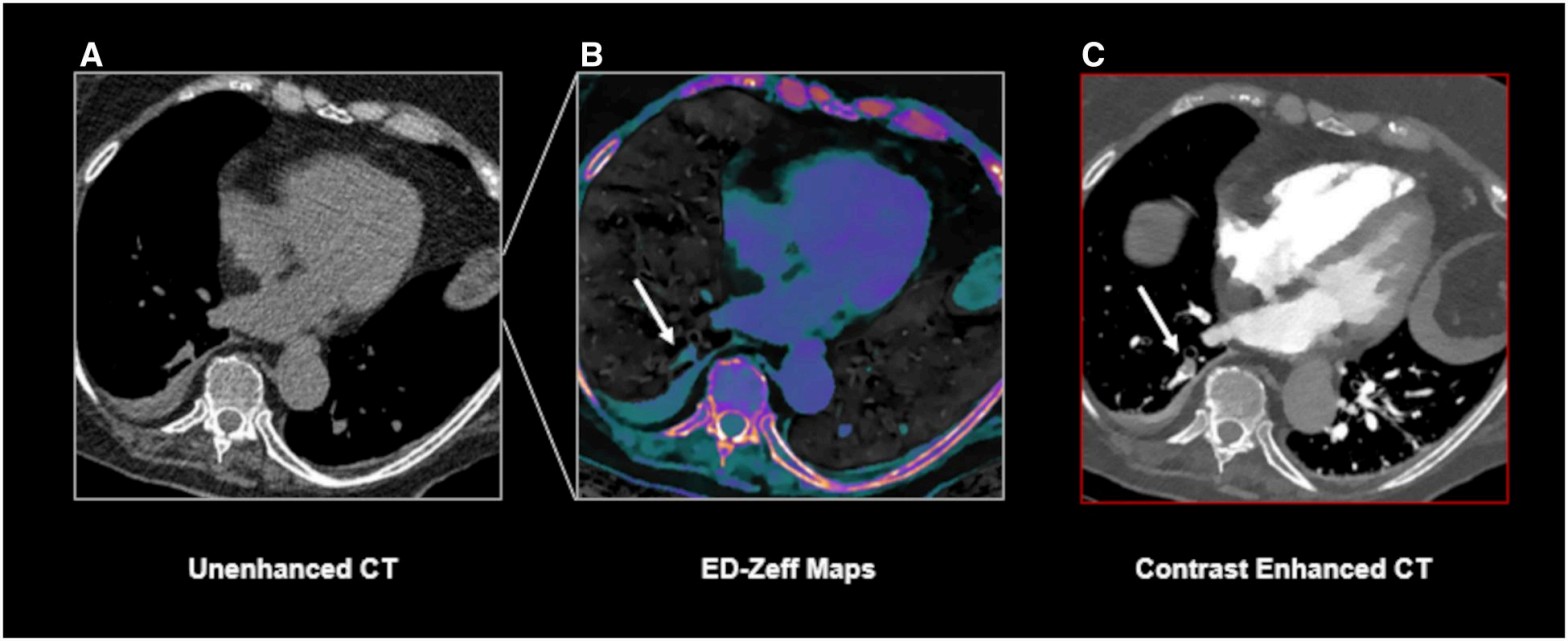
A false-negative case of PE identified using the ED-Zeff (electron density-Z-effective) map. A 69-year-old male patient presented to our department with dyspnea. Unenhanced CT scan of the chest (A) shows no relevant findings. ED-Zeff map (B), derived from the unenhanced CT scan, does not show any luminal difference of signal within a right segmental artery (arrow). The subsequently performed CECT (contrast-enhanced CT) revealed the presence of a sub-segmental pulmonary embolus.
